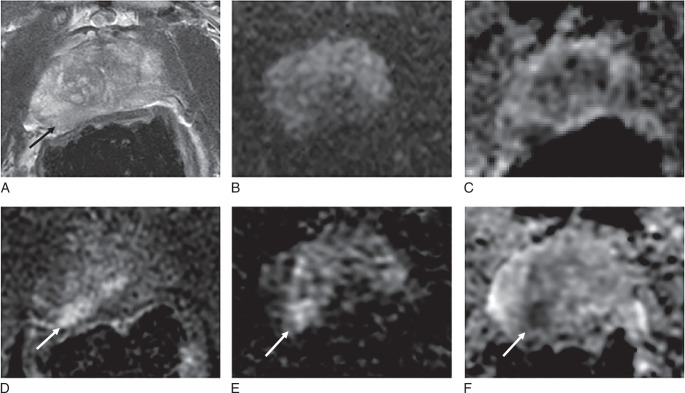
Example of a false-negative case with nondiagnostic supine DWI. Seventy-six-year-old patient, PSA 11.21 ng/mL. A–D, Supine imaging shows wedge-shaped mild low T2 signal in the right mid/apex PZ, PI-RADS 2 (arrow, A), poor quality DWI, PI-QUAL 1/4 (B, C), with marked focal early enhancement on DCE (arrow, D). E and F, Prone DWI is diagnostic and shows marked restricted diffusion, PI-RADS score 4. PI-RADS score 2 using supine imaging alone, with nondiagnostic DWI, but PI-RADS score 4 when using prone DWI. Targeted biopsy shows Gleason 3+4=7 in 1/3 cores with 5 mm max tumor length.
